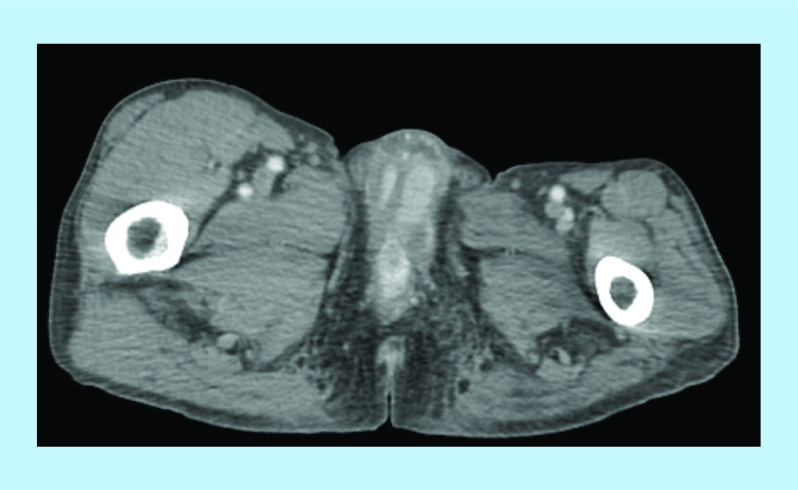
Pelvic computed tomography showing poorly limited hypodense lesions of corpora cavernosa.
